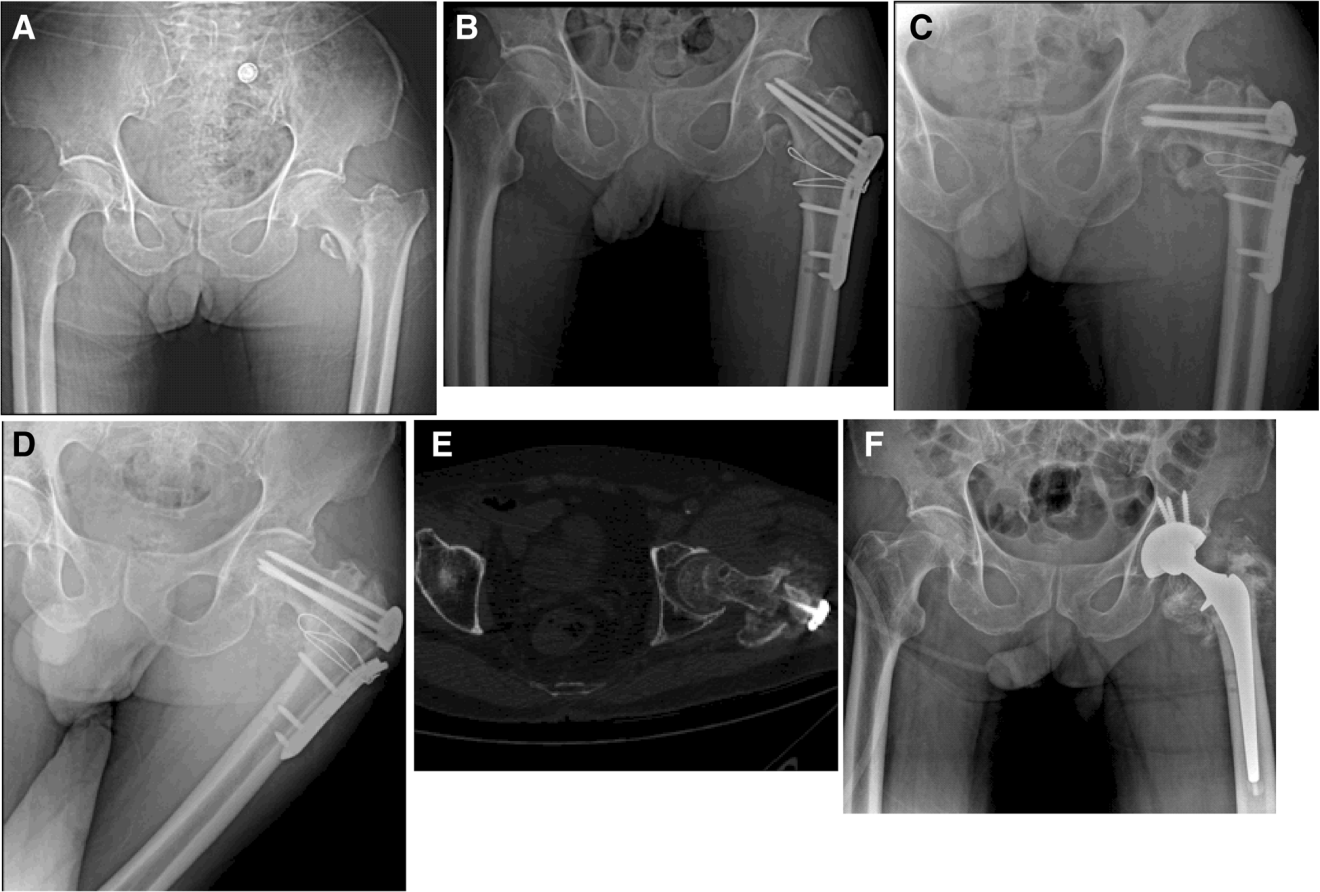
A 75-year-old male with AO/OTA type 31A2.2 experienced plate breakage. a Radiograph after injury. b Postoperative radiograph showing good reduction and fixation with PFLP (1 week after surgery). c Plate breakage and varus collapse 16 weeks after surgery. d, e Postoperative radiograph and computed tomography (CT) showing nonunion (18 weeks after surgery). f Revision surgery of THA
